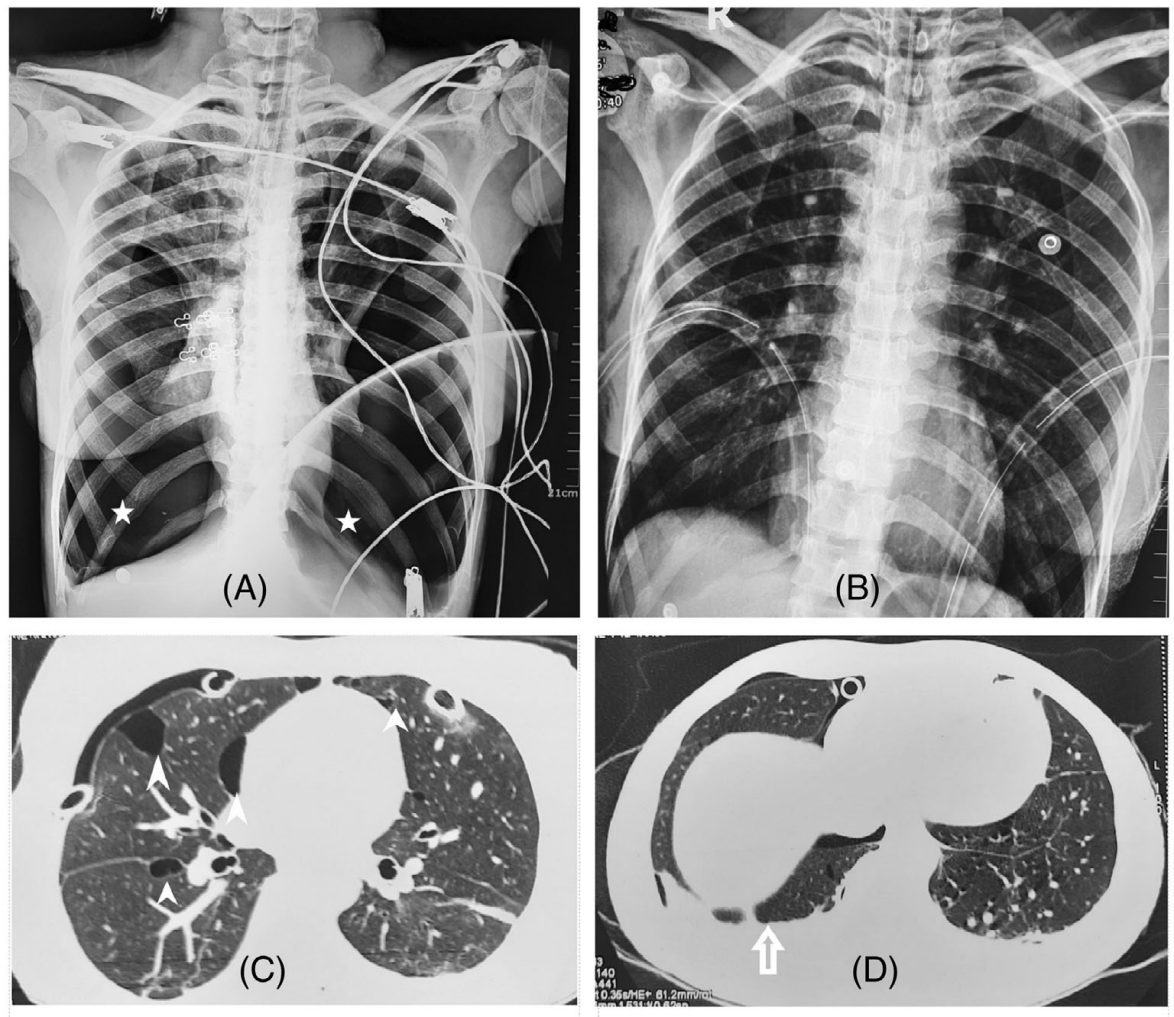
The chest x‐ray showing bilateral pneumothorax in (A) (white asterixis) before the chest drains insertion. The resolution of the pneumothorax following chest drain insertion in (B). The axial views of computed tomographic imaging showing bilateral thin‐walled pulmonary cysts in (C) (white arrowheads) and thin pleural effusion in (D) (white arrow).
